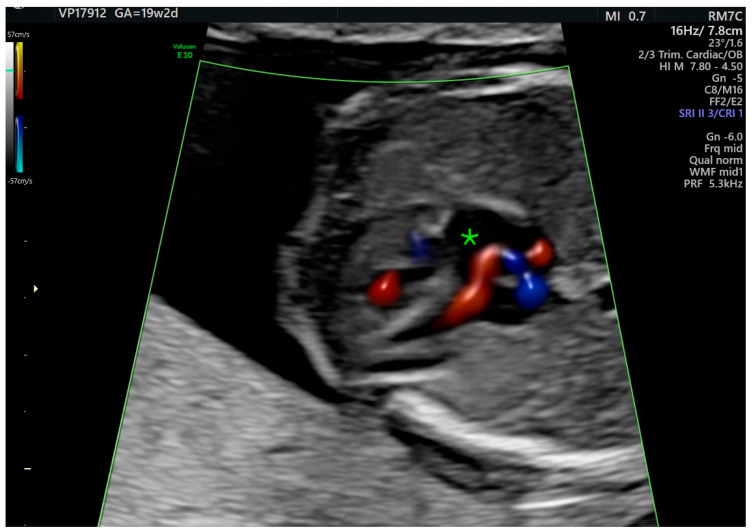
Complete AVSD in a Down syndrome fetus of 19 weeks + 2 days: color Doppler US shows aortic vascular efflux (star) which partially overlap the atrial blood flow.
